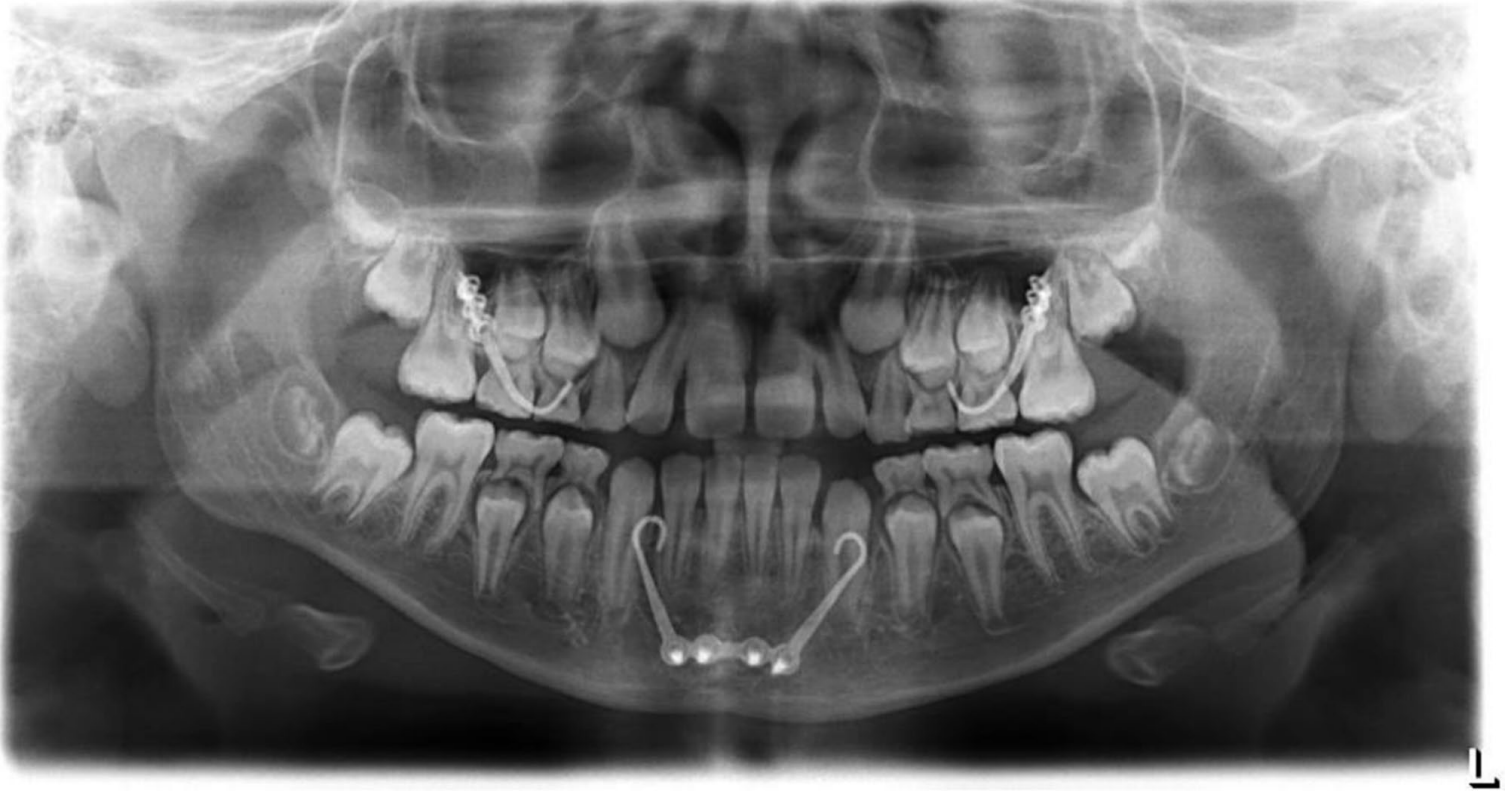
Panoramic radiograph with inserted bone anchors in upper and lower jaw on both sides
